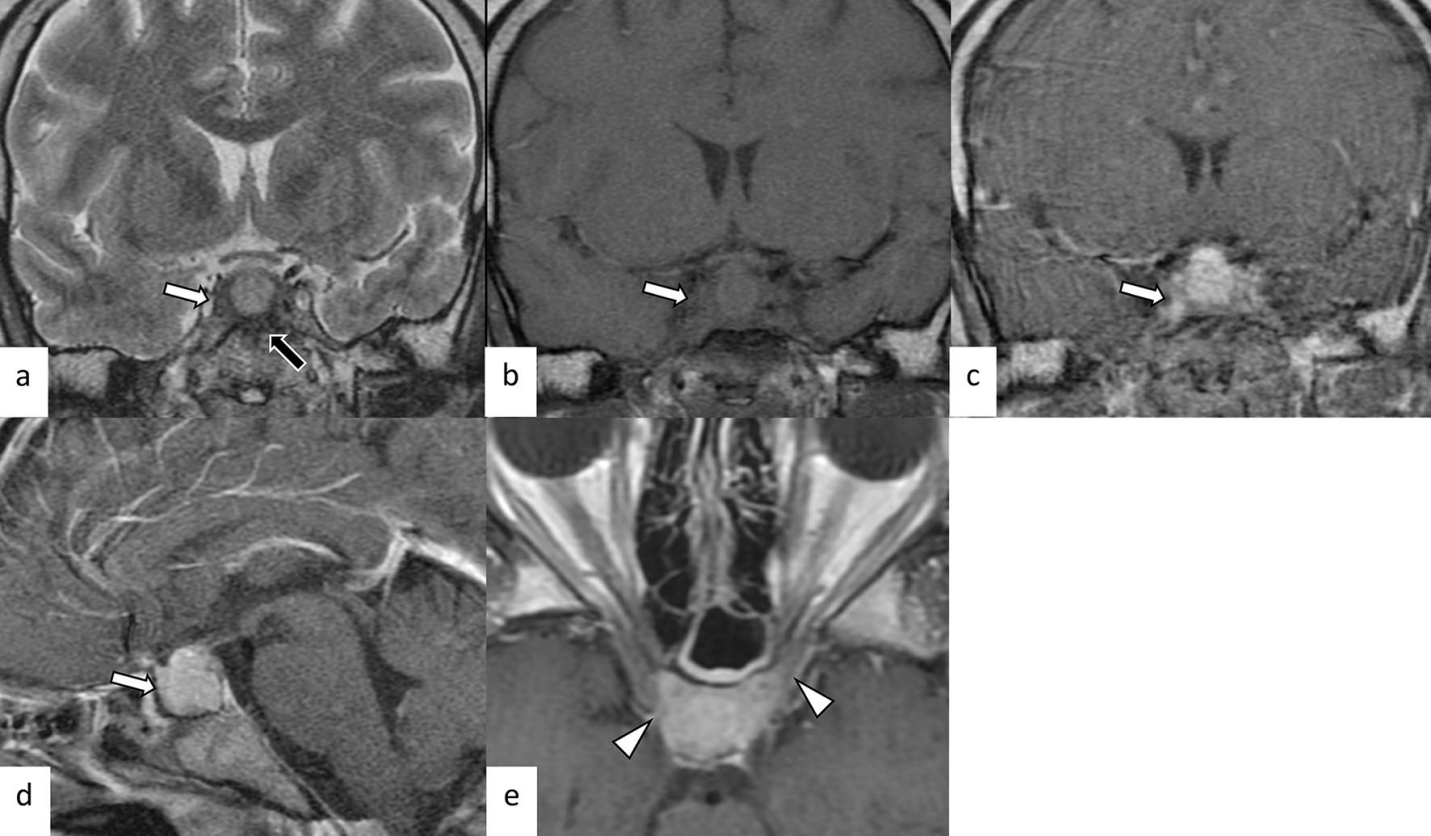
A 29-year-old man with primary granulomatous hypophysitis. Artifacts due to the patient’s body motion are observed. An enlarged pituitary gland shows heterogeneous hyperintensity on T2-weighted coronal image (a, white arrow), isointensity on T1-weighted coronal image (b, white arrow), and homogeneous contrast enhancement on post-contrast T1-weighted coronal and sagittal images (c, d, white arrows). Extension into bilateral cavernous sinus is observed (e, arrowheads). T2-weighted hypointensity areas around the enlarged pituitary gland are found, suggestive of granulomatous/fibrous inflammation (a, black arrow)
